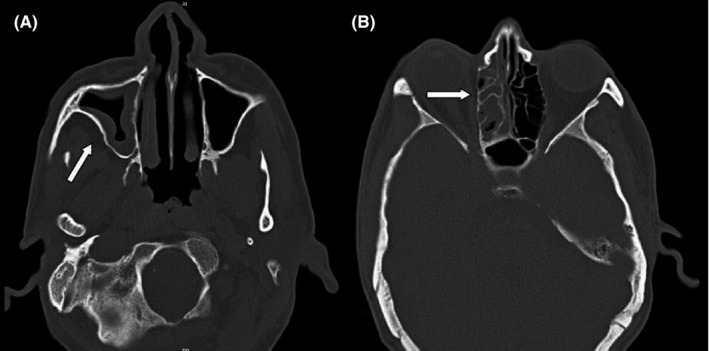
Computed tomography (CT) scans of the sinuses in Invasive *Aspergillus* rhinosinusitis. Thickness in the maxillary, sphenoid, and frontal sinuses (A), opacification in the right ethmoid sinus (B)
